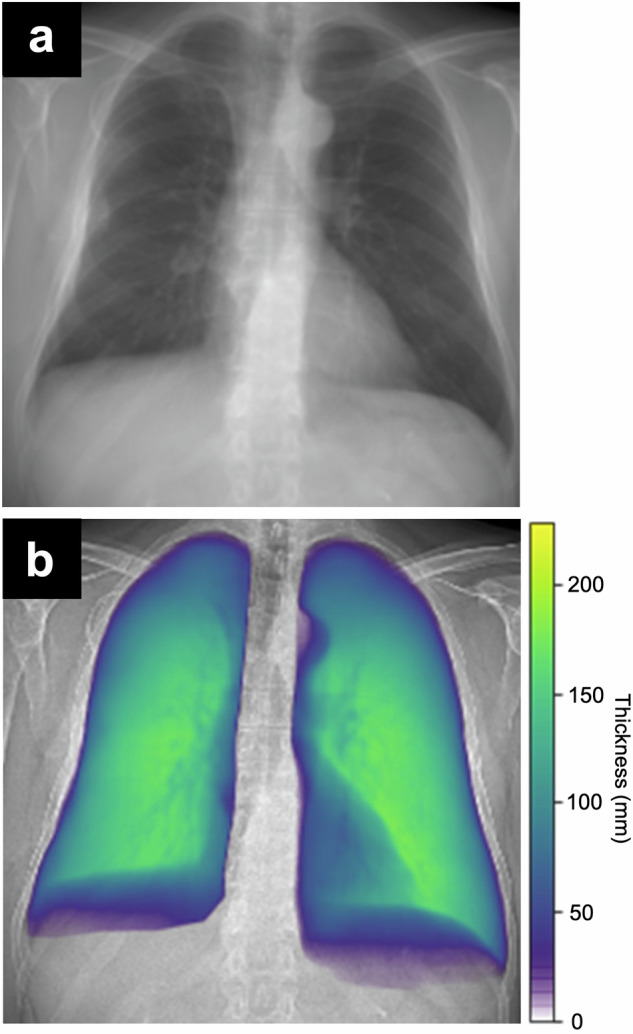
Example attenuation-based radiograph acquired with the dark-field scanner (a) and computed lung thickness map (b)
